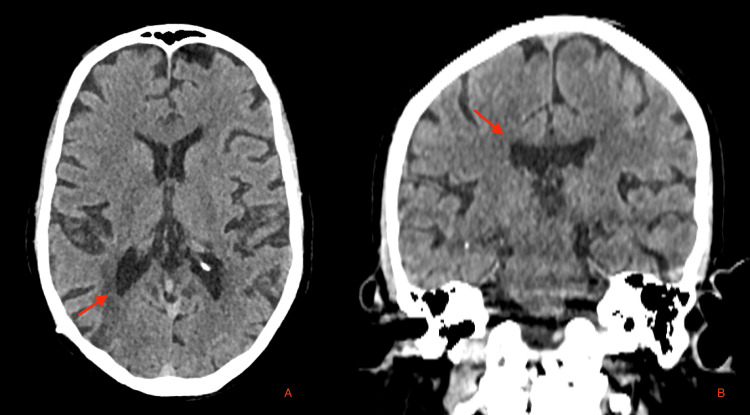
One-year follow-up cranial CT images showing maintained complete resolution of the previously observed intraventricular pneumocephalus and left temporal encephalomalacia. (A) Axial and (B) coronal reconstructions demonstrate normal ventricular morphology and no evidence of intracranial air (red arrow). CT: computed tomography
